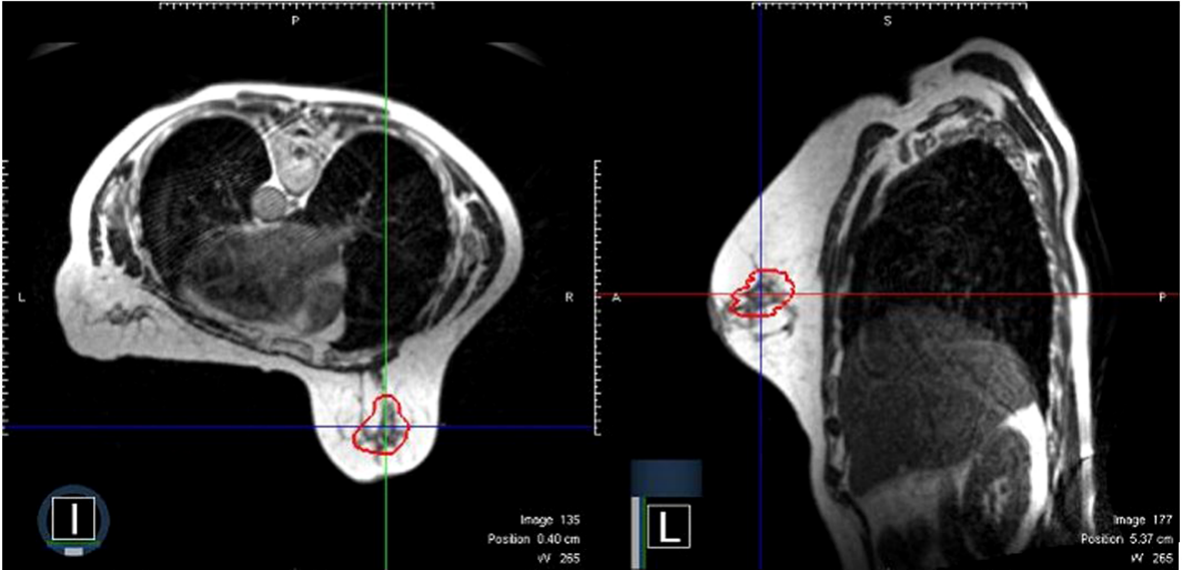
A representative surgical cavity target volume seen from an MRI image acquired on-table on the day of radiation treatment. The MRI scans in both the axial and sagittal planes are shown. The surgical cavity is outlined in red.
